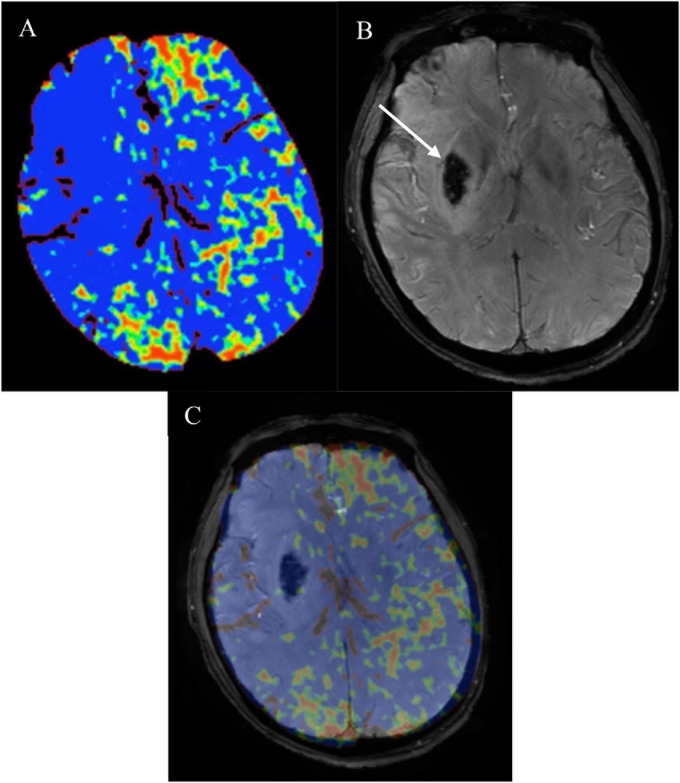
(A) CT perfusion permeability surface (PS) map, follow-up (B) susceptibility-weighted imaging (SWI) in the same patient as in Figure 1B and (C) co-registered map. Obviously increased (A) PS on the affected side compared with the contralateral normal side. Follow-up (B) SWI showed hemorrhagic transformation (HT) (white arrow) which belonged to parenchymal hematoma (PH) 2. PS map and follow-up SWI image were co-registered automatically using OpenCV 2.4.9. Then the regions of interest (ROIs) were drawn within the HT region and the corresponding contralateral region. Relative PS were calculated by normalizing the values in the HT region with those in the contralateral mirror region. PS, permeability surface; SWI, susceptibility-weighted imaging; HT, hemorrhagic transformation; PH, parenchymal hematoma; ROIs, regions of interest.
